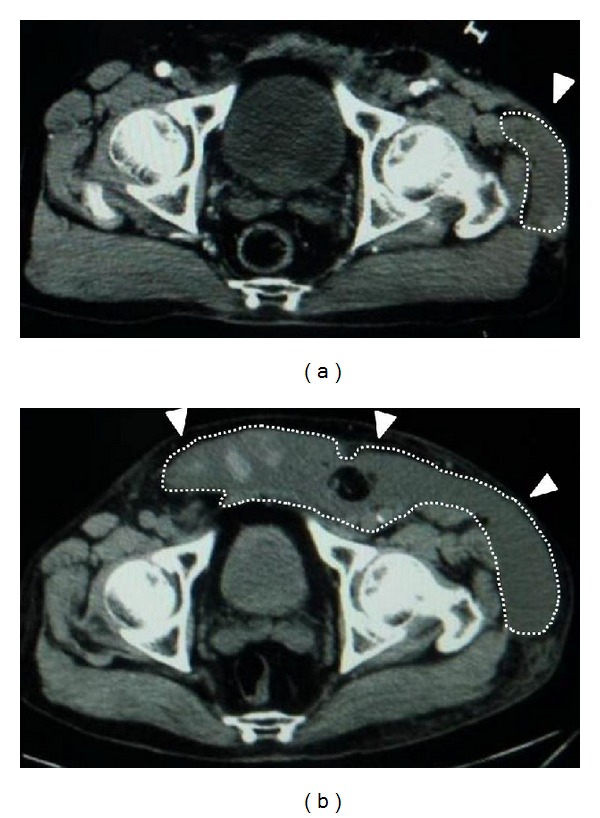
CT axial image around the pelvis. (a) At the initial evaluation, a narrow low-density area was found around the left greater trochanter and no fracture was evident. (b) Seven days after the trauma, the low-density area extended from the lower abdomen to the lateral thigh.
